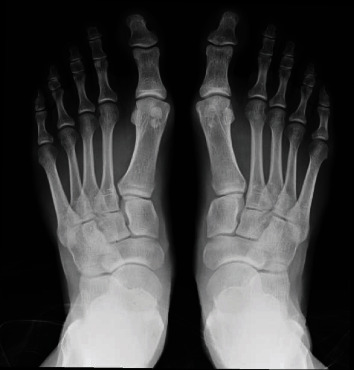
X-ray of the right and left feet demonstrating bilateral bipartite sesamoid bone and absence of other alterations.
